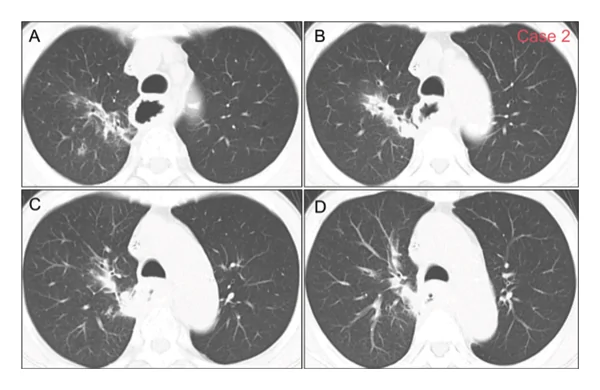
Case 2 patient’s chest CT showed immune‐related pneumonitis in the right lung. Chest CT scans (A) and (B) revealed Grade 2 immune‐related pneumonitis in the right lung following a cycle of toripalimab combined with chemotherapy. Scans (C) and (D) indicated improvement of the lesion compared to the previous scan, suggesting Grade 1 immune‐related pneumonitis after temporarily discontinuing toripalimab.
